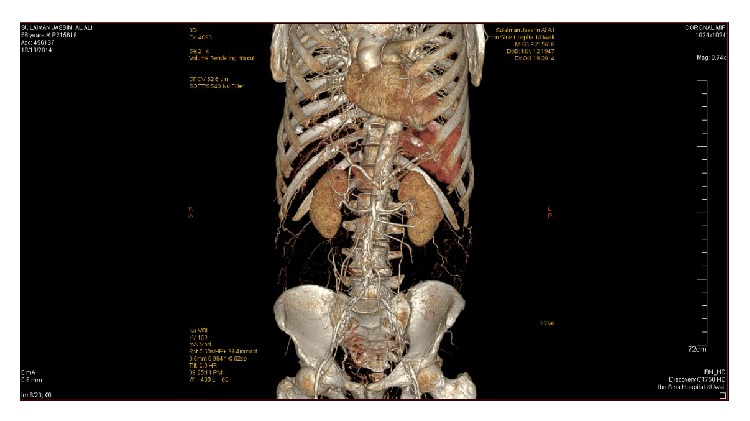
Thoracoabdominal computed tomography with angiographic study showing thrombosis of the distal aorta and both common iliac arteries (coronal view).
